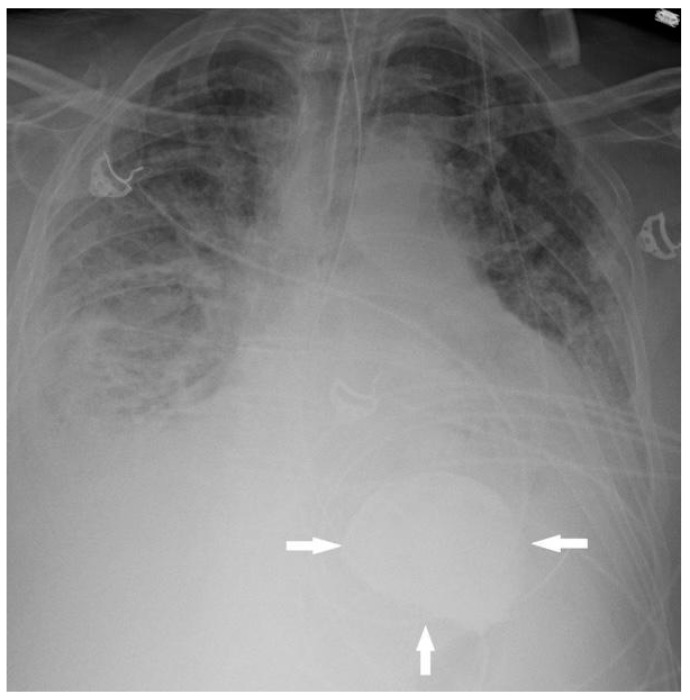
A 61-year-old man with shortness of breath. Chest radiograph showing hyperattenuating contents in the lumen of the stomach (arrows). After consultation with the intensive care physician caring for the patient, it was found that the patient was being treated for hyperkalemia with SZC, accounting for this finding.
